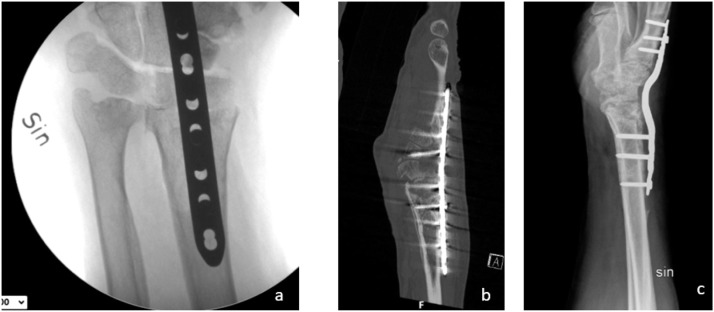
Radiographs after first and second radiocarpal arthrodeses: a) perioperative radiographs after radiocarpal arthrodesis; b) no bony union one year after the first procedure; c) no bony union one year after the second procedure.
